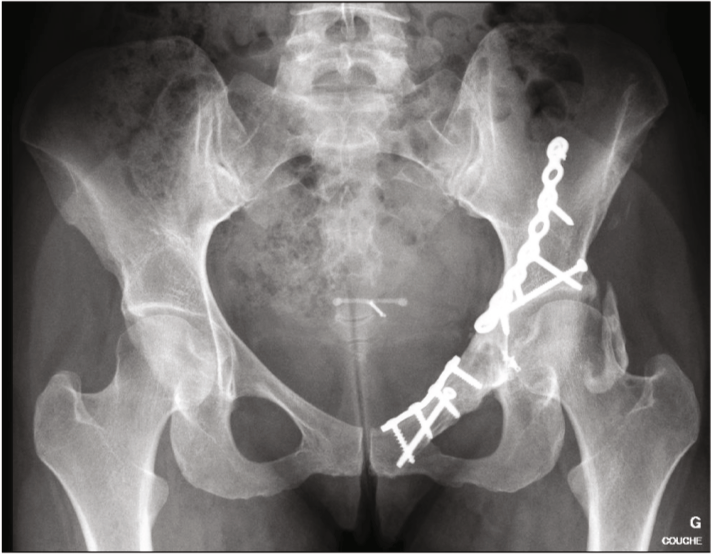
Plain film at 4 years of follow-up showing healed osteotomy sites without signs of femoral head avascular necrosis or osteoarthritis. Note the presence of heterotopic ossification.
